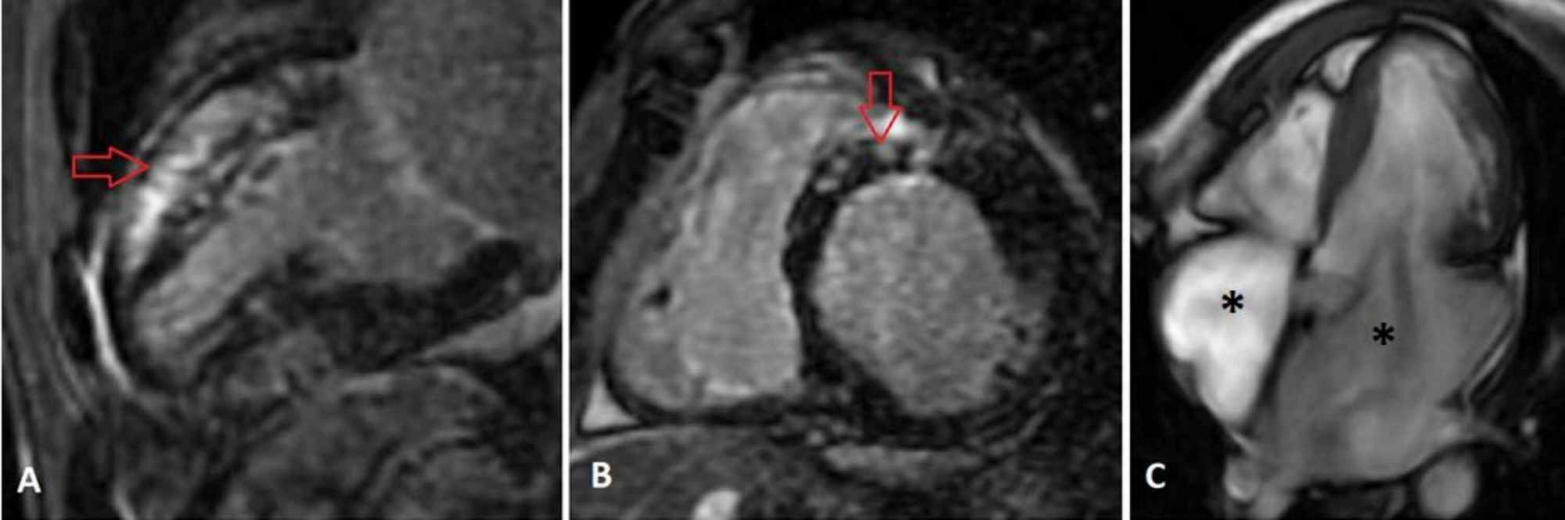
Cardiac magnetic resonance imaging showing abnormal delayed enhancement at subepicardial and mid-myocardial anterior and anteroseptal walls (red arrows), two chamber (A) and short axis views (B). Steady state free precession (SSFP) showing bi-atrial enlargement (black asterisks) (C)
